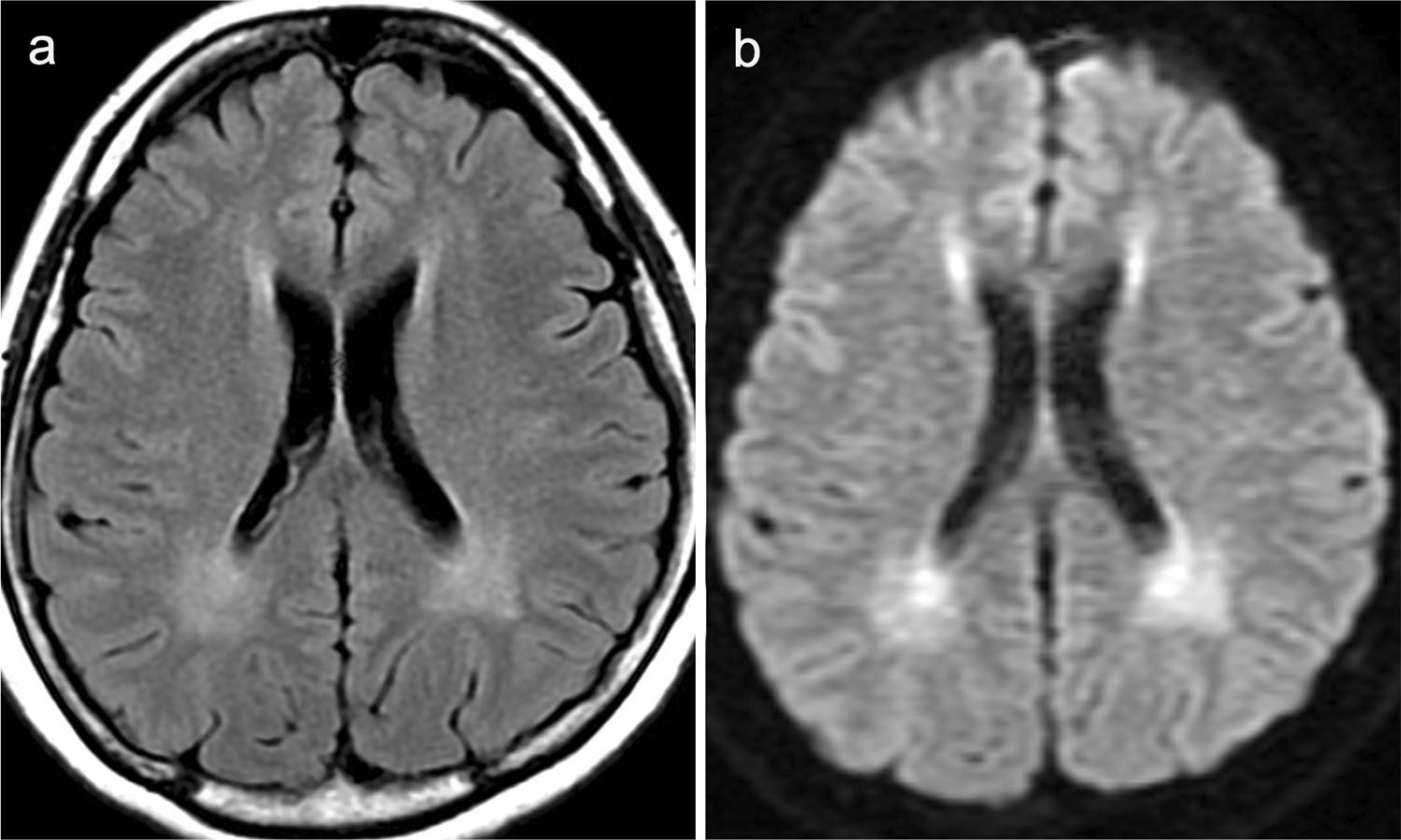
A 32-year-old, female patient with phenylketonuria (PKU). a FLAIR image showed increased signal intensity in the periventricular white matter. b DWI showed high-signal intensity in the periventricular white matter, indicating restricted diffusion and intramyelinic edema
